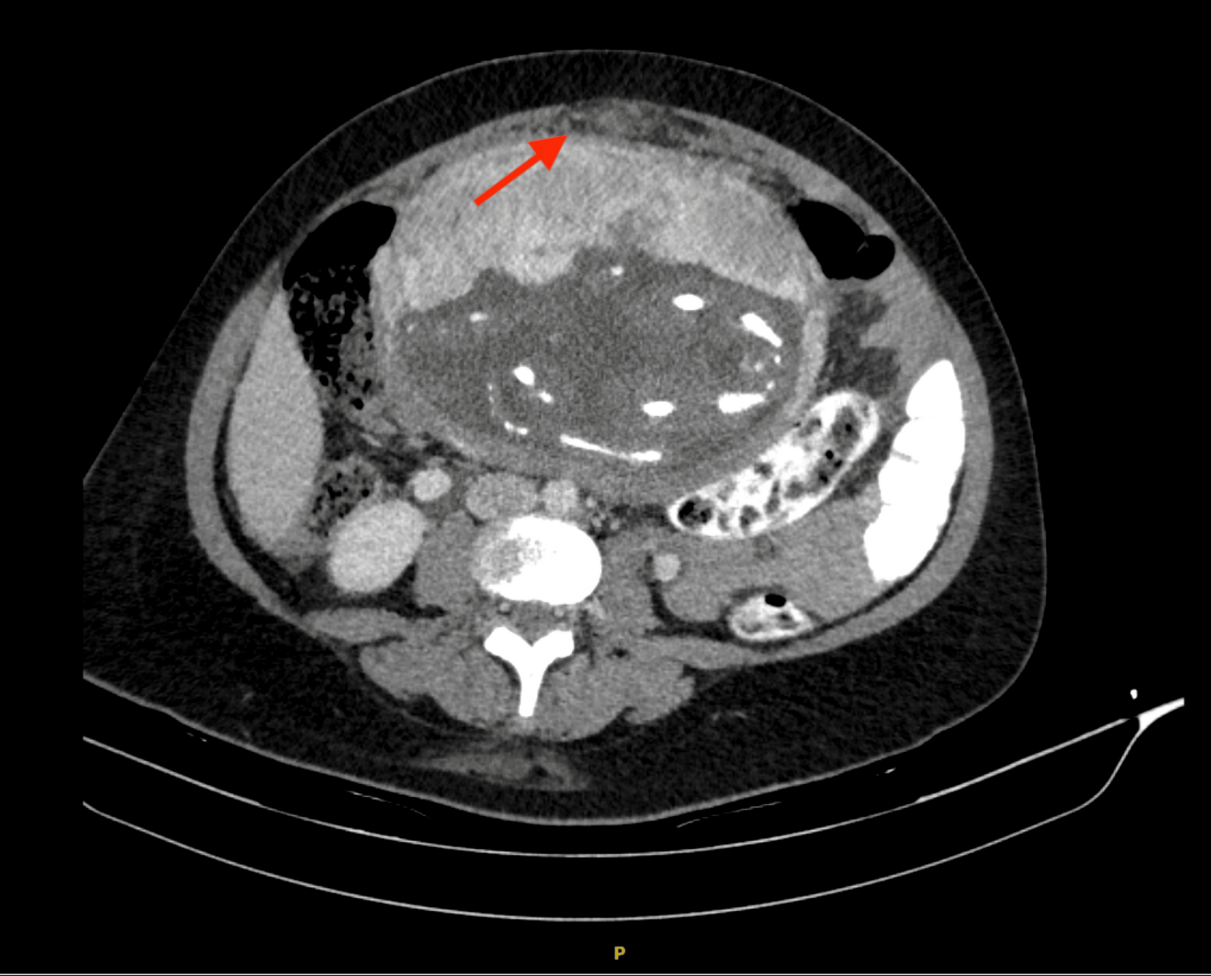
The initial CT scan demonstrated evidence of omental thickening, and the foetus within the uterus was visualised
